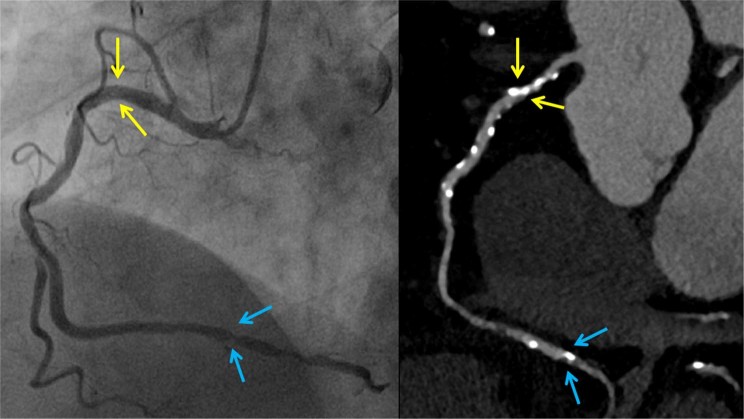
False positive finding. Overestimated (false positive) proximal right coronary artery (RCA) stenosis on coronary computed tomographic angiography (CCTA) under 100 kVp/150 mAs in a 66-year-old man (effective radiation dose, 1.64 mSv; BMI, 26.1 kg/m^2^; mean heart rate, 63 bpm). Invasive coronary angiography (ICA) of the RCA confirmed the insignificant stenosis (a). Curved multiplanar reformation of the RCA shows significant stenosis in the proximal segment caused by a calcified plaque (b), although this was a false positive finding as compared to the ICA. In the same vessel, distal right coronary stenosis was concordantly detected (true positive) via CCTA and ICA.
